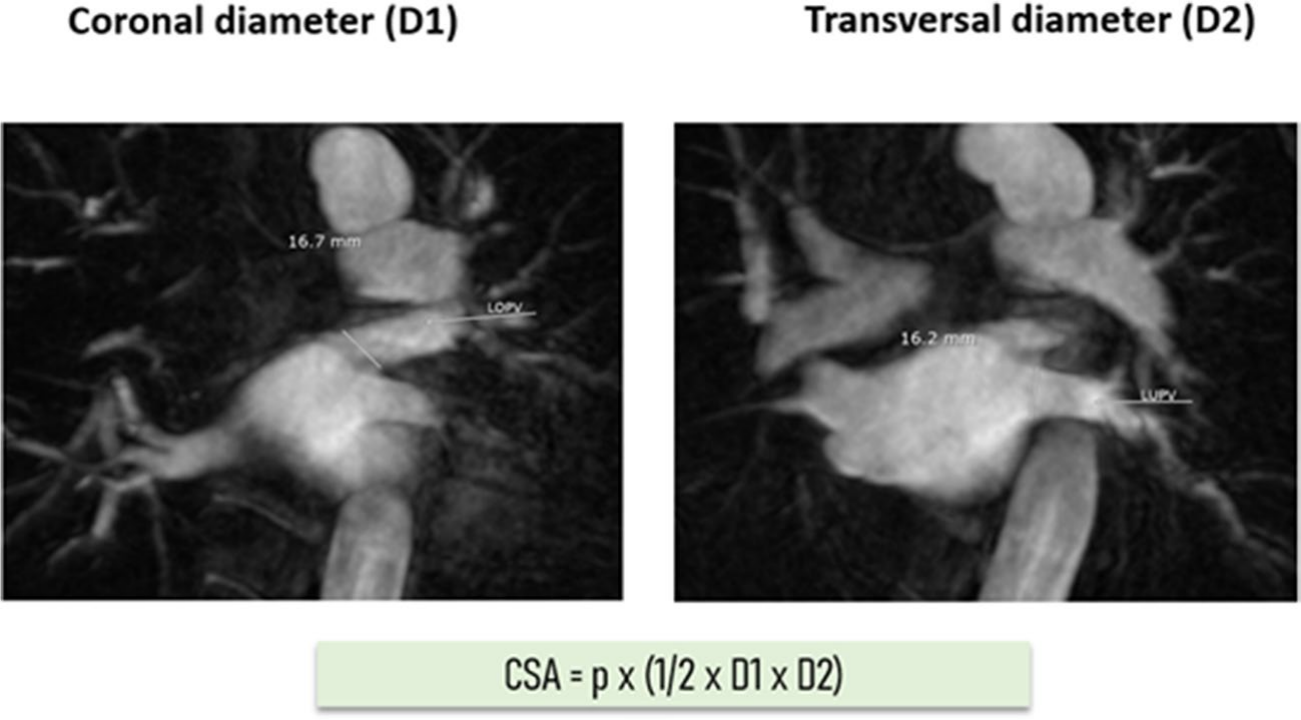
MRI-based measurement of the PVs’ coronal (D1) and transversal diameter (D2). MRI, magnet-resonance imaging; PV, pulmonary vein; CSA, cross-sectional area; D1, coronal diameter; D2, transversal diameter; *p*, Pi-number, 3.14; LOPV, left superior pulmonary vein; LUPV, left inferior pulmonary vein
